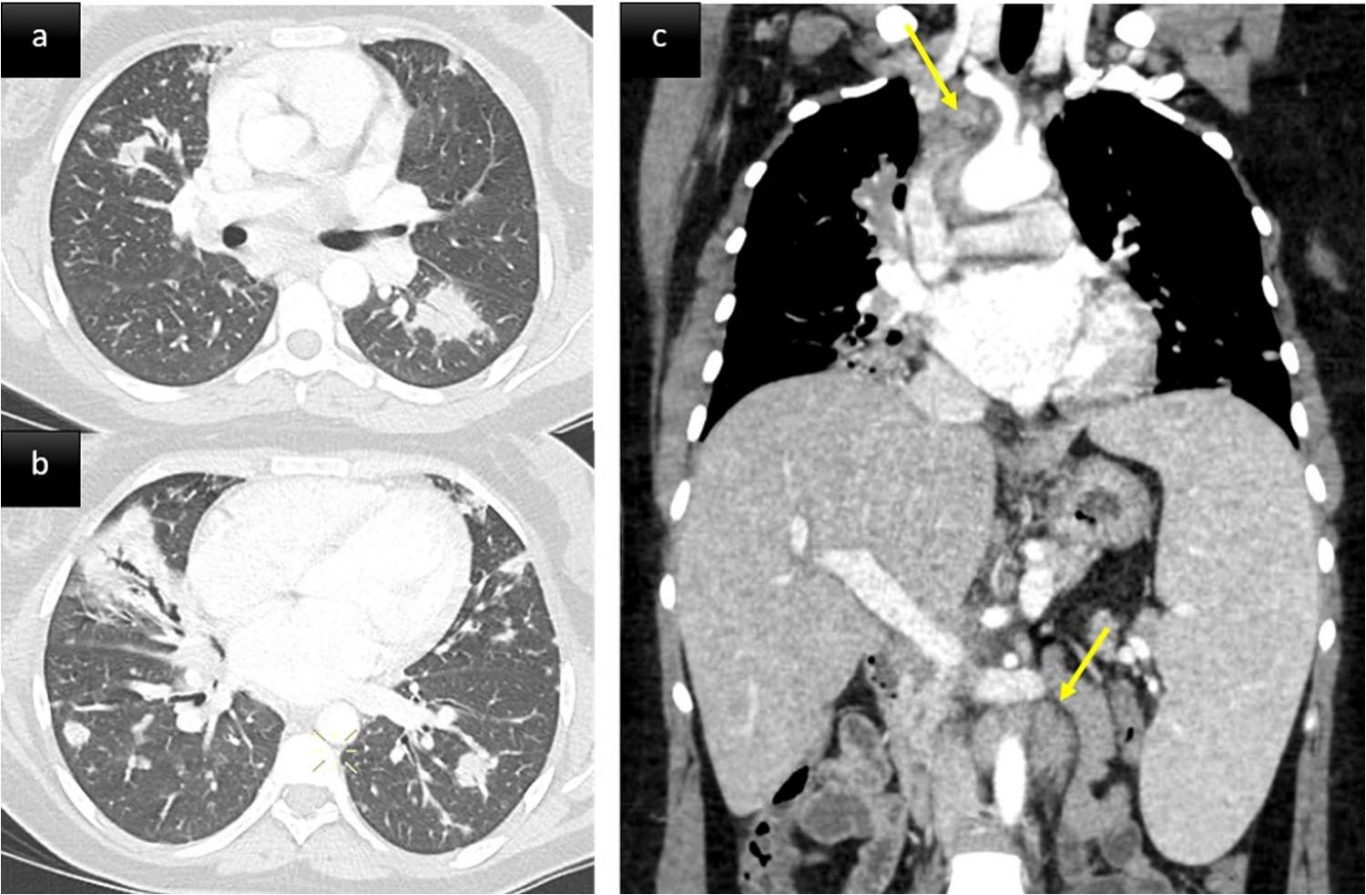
A middle aged woman with CVID. Post contrast thoraco-abdominal CT scan, axial parenchymal (a, b) and coronal soft tissue (c) images. Lower zone predominant broncho centric nodules and airspace consolidation consistent with granulomatous-lymphocytic interstitial lung disease (GLILD). The patient also presented with splenomegaly, mediastinal and retroperitoneal lymphadenopathy (arrows)
